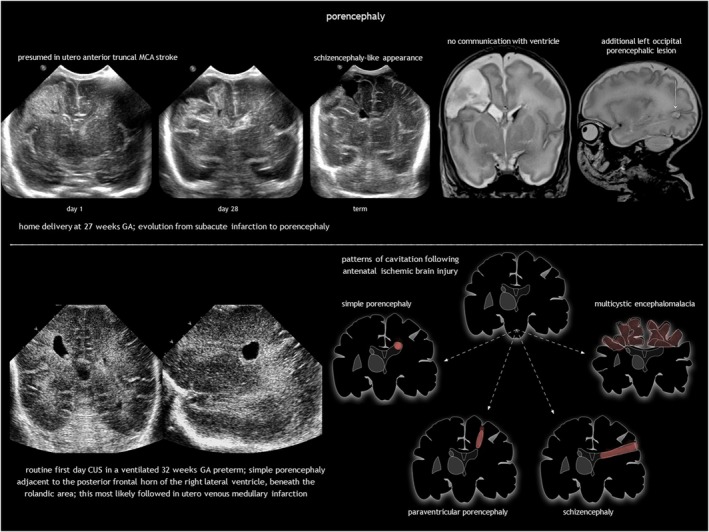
Porencephaly. Abbreviations: CUS, cranial ultrasonography; MCA, middle cerebral artery.
